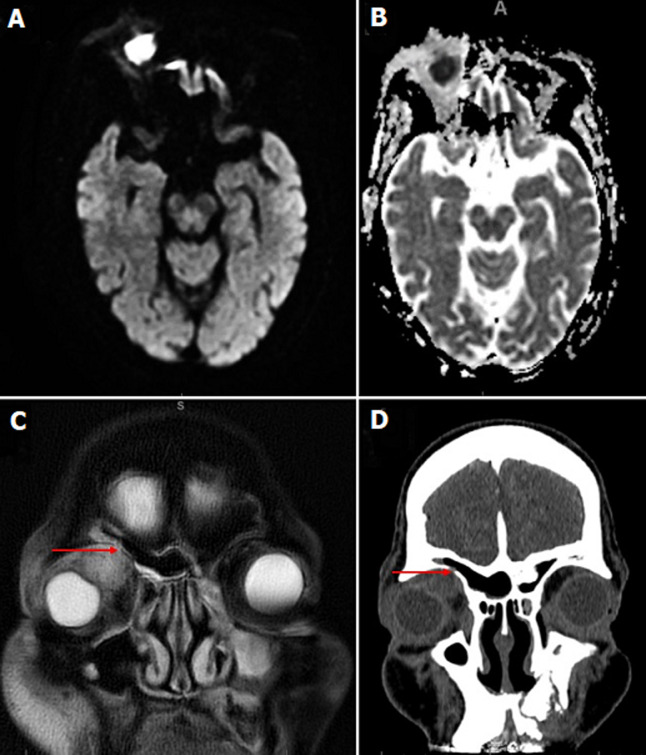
A) axial diffusion-weighted imaging MRI of the orbits without contrast; B) axial MRI image with companion apparent diffusion coefficient; C) coronal T2 fat suppressed MRI; D) coronal CT scan showing a superomedial orbital mass with restricted diffusion, and trace of fluid tracking to the frontal sinus (red arrow)
